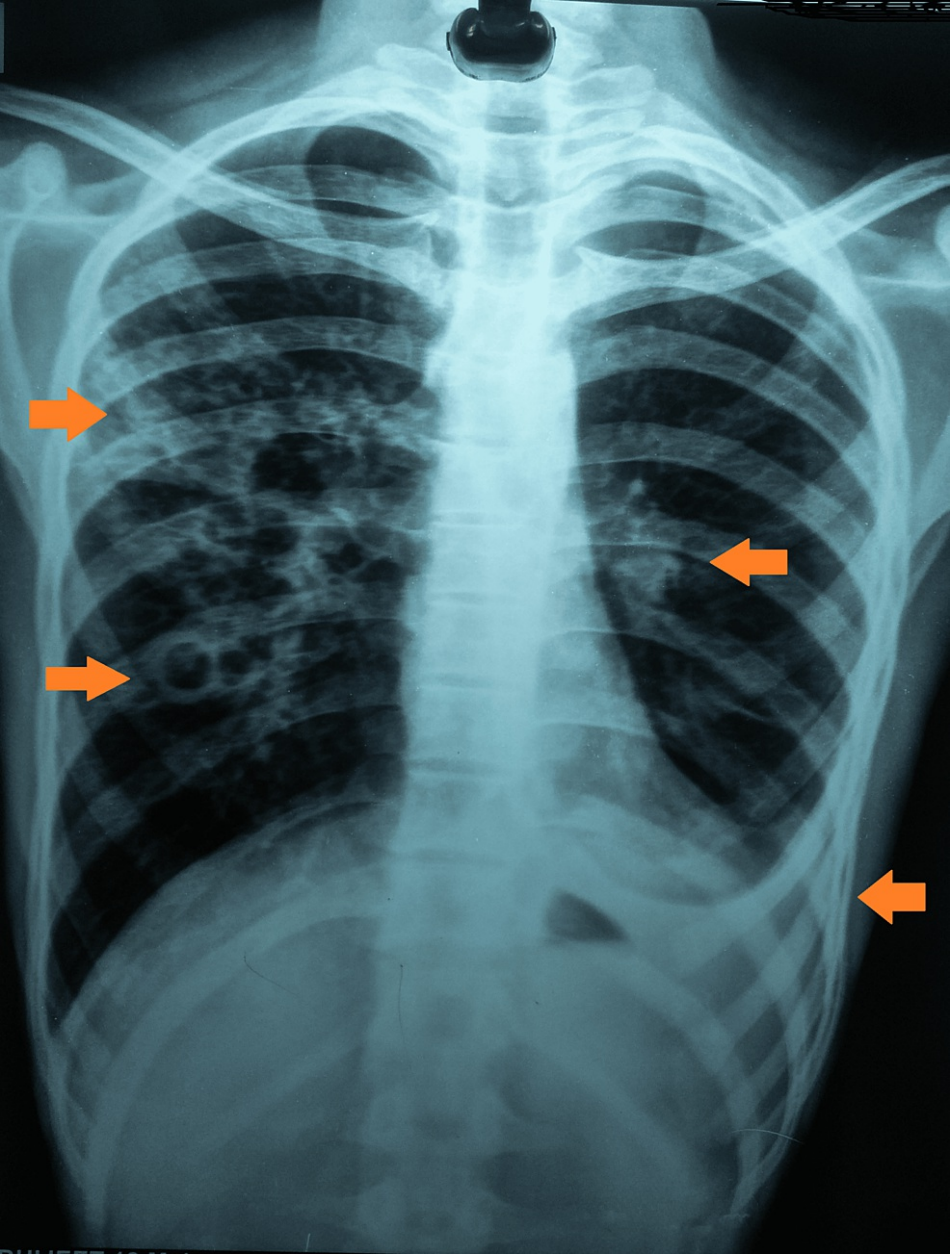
Chest radiograph (P-A) view showing bilateral involvement of the lungs P-A: Posteroanterior
